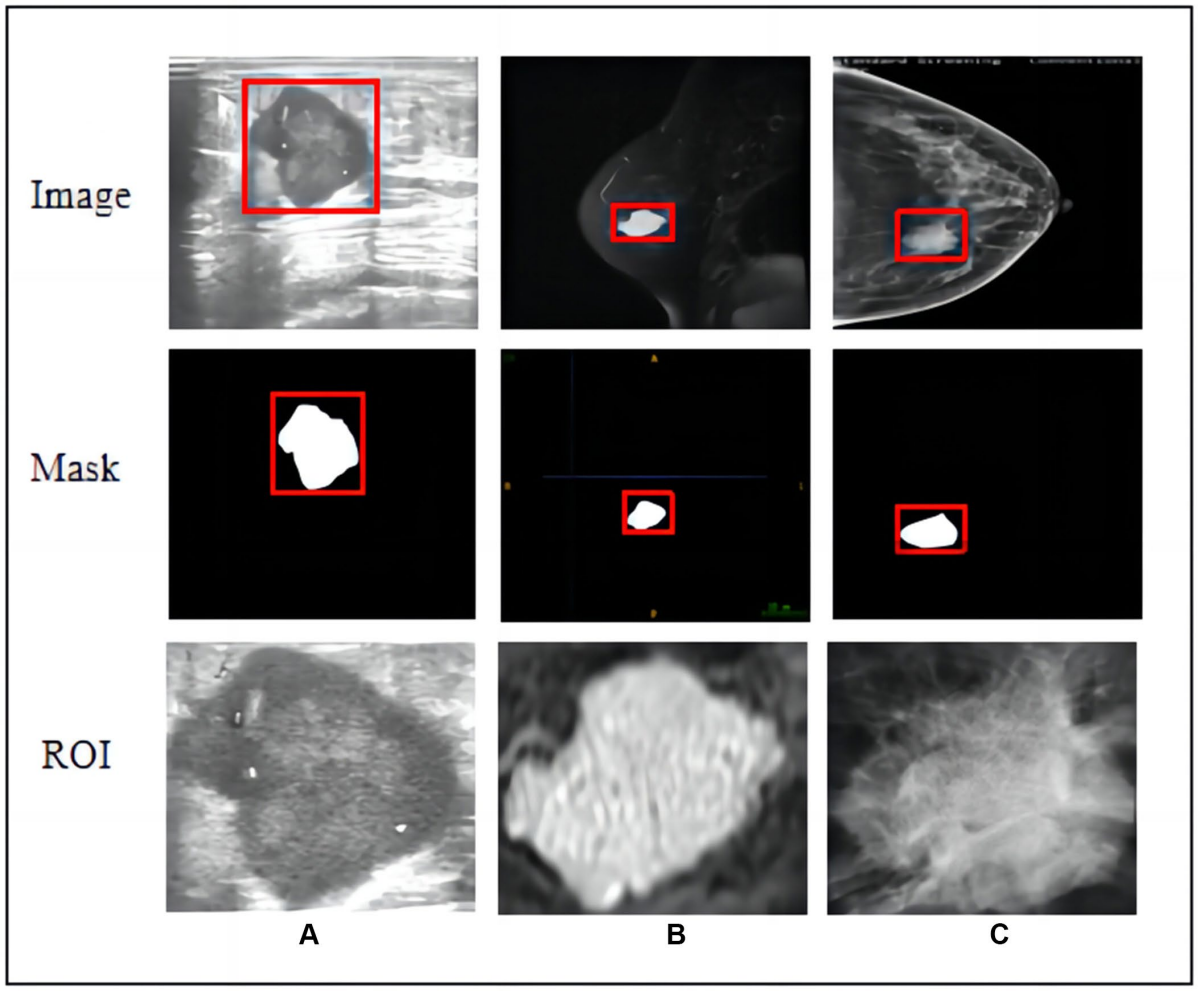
Raw images, hand-crafted masks, and cropped ROIs of three modal images. (A) Ultrasound, (B) T2-weighted magnetic resonance imaging, and (C) mammography (MG) craniocaudal view.
